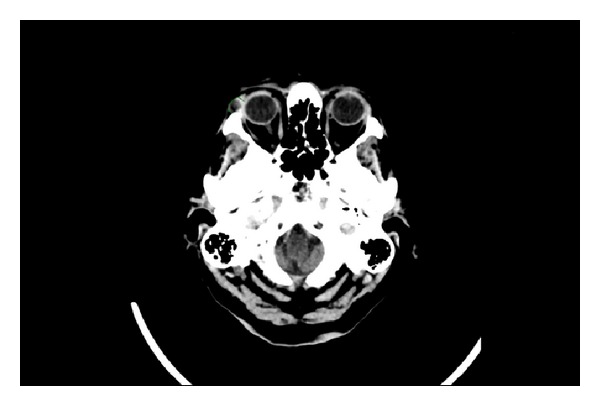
Axial view of CT scan showing a right supraorbital mass prior to surgery.
